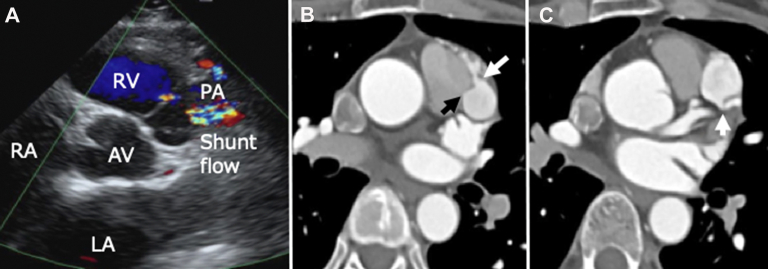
(A) The Doppler color image showed a continuous shunt flow to the main pulmonary artery (PA) on echocardiography. Enhanced computed tomography showed the aneurysm connecting to the (B) main pulmonary artery (black arrow) and branches of the right coronary artery (white arrow) and (C) left anterior descending coronary artery (arrow). (AV, aortic valve; LA, left atrium; RA, right atrium; RV, right ventricle.)
